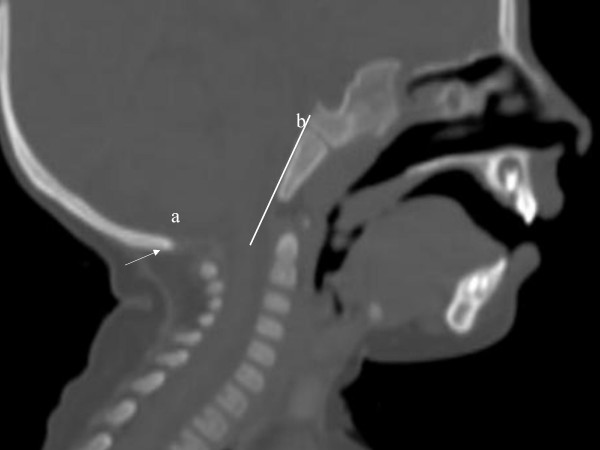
3D sagittal CT scan; Agenesis of the posterior arch of the atlas (arrow-a). Arrow (b) notes the Wachenheim clivus line, which is drawn along the posterior aspect of the clivus toward the odontoid process; in our patient the line does not intersect or is it tangential to the odontoid process. The latter confirms the existence of progressive craniocervical abnormality.
